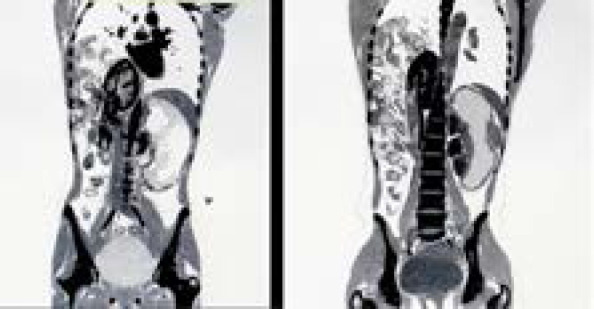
Left CT showing total eventration of right hemidiaphragm with segments of the bowel loop and right kidney in the thoracic cavity. Right CT showing functional right ectopic kidney.
